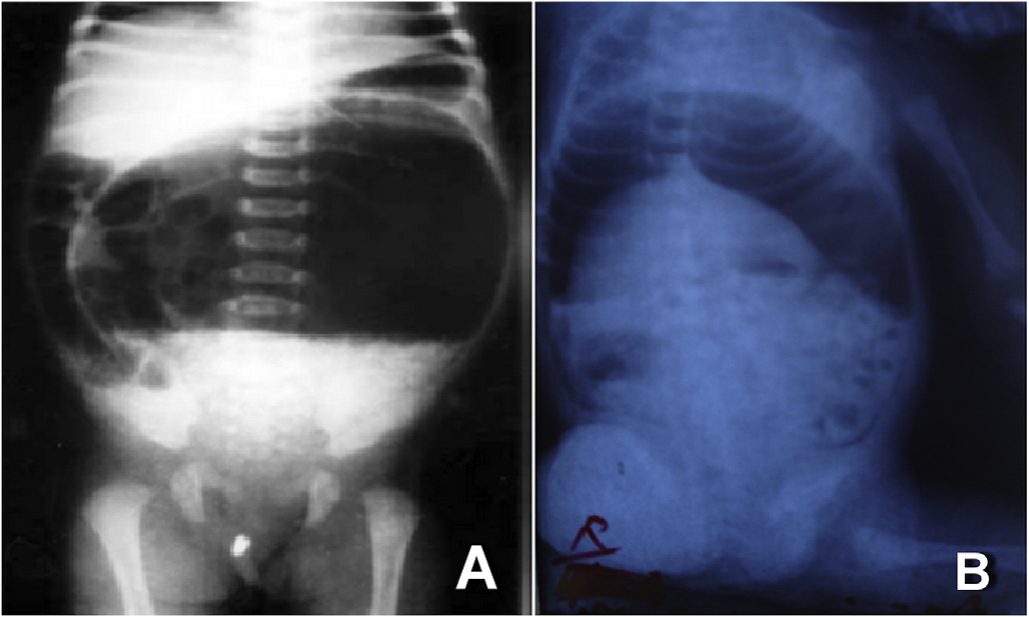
Plain abdominal X-ray taken preoperatively showing a large air fluid level(A) and free air under both doms of the diaphragms (B).
